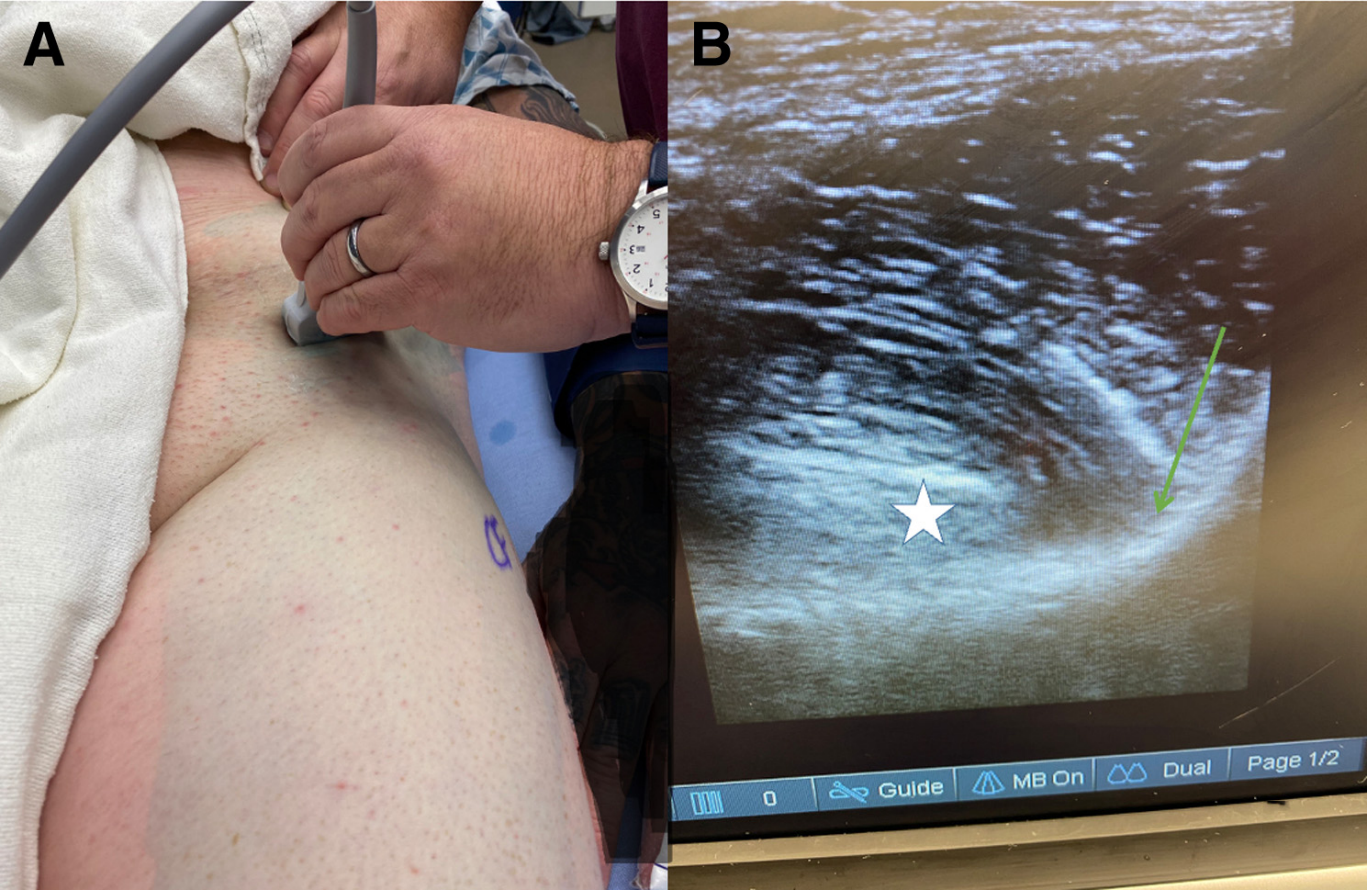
(A) Patient is in the supine position undergoing left PENG block. The ultrasound probe is over the anterior superior iliac spine and turned 45° to be in line with the iliopectineal eminence (IPE). (B) Needle is inserted in plane between the IPE and psoas tendon. Green arrow shows the IPE; star shows the psoas tendon. (PENG, pericapsular nerve group.)
